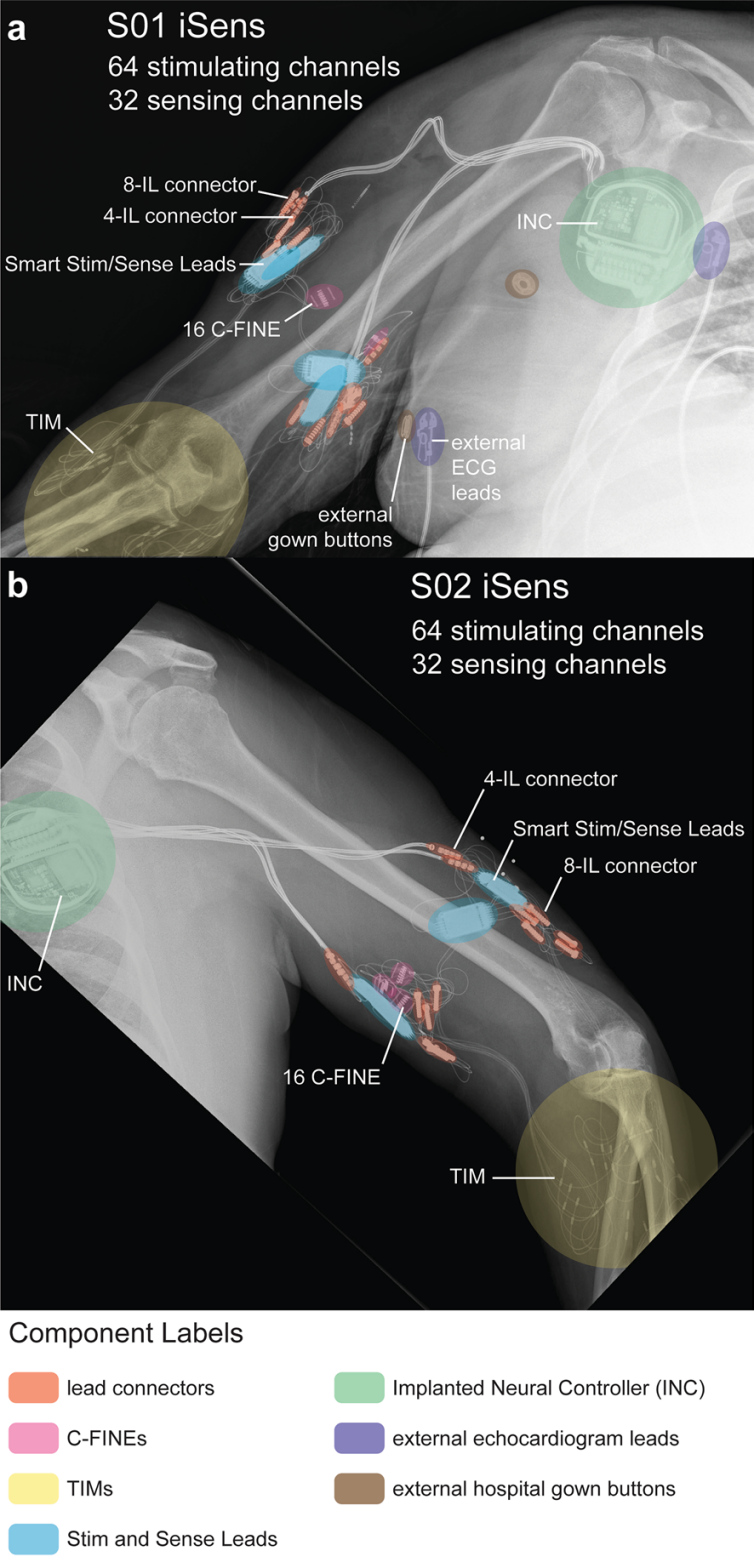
iSens® X-rays. X-rays showing iSens® implanted components in a S01 and b S02. To improve lead organization compared to S01’s original surgery, implanted device components and connectors in S02’s system were deliberately routed along the same direction, and excess lead length was intentionally organized to reduce bulk
